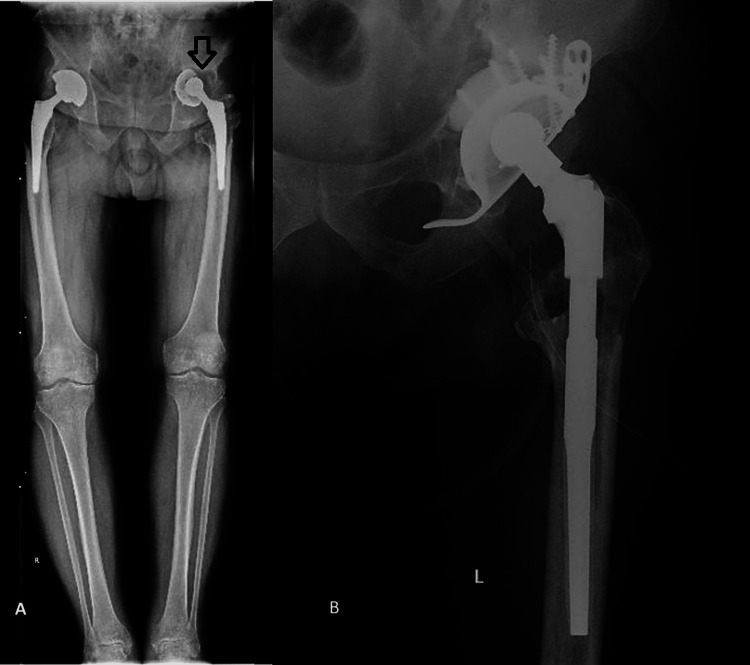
A. Preoperative full-leg X-ray. B. Post-operative left hip X-ray.
